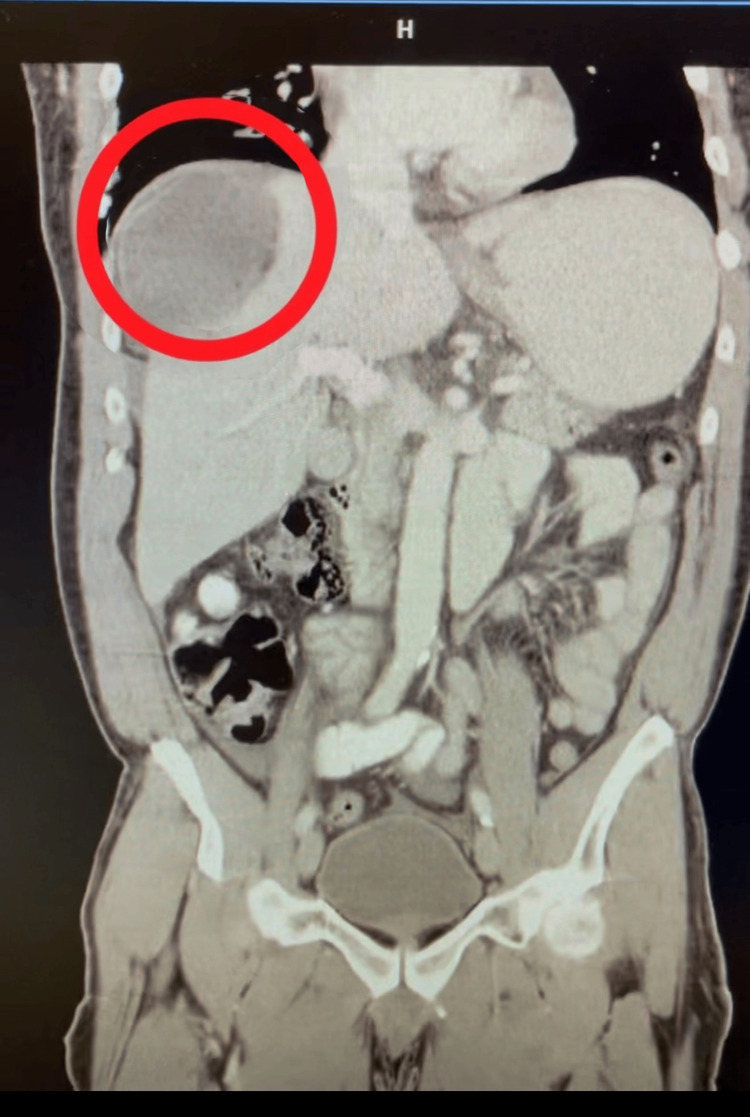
Repeat CT scan of the abdomen and pelvis in coronal view. There is a well-circumscribed, rounded structure in the right upper quadrant (circled in red) with predominantly fluid attenuation. A small amount of intraluminal gas is seen within the superior aspect of the structure, suggesting communication with bowel or entrapped air. The lesion appears to displace adjacent small bowel loops inferiorly, consistent with a localized mass effect. Surrounding peritoneal fluid is noted, suggestive of reactive or inflammatory changes. No evidence of free intraperitoneal air is identified. Liver, spleen, pancreas, and kidneys appear unremarkable in this section.
